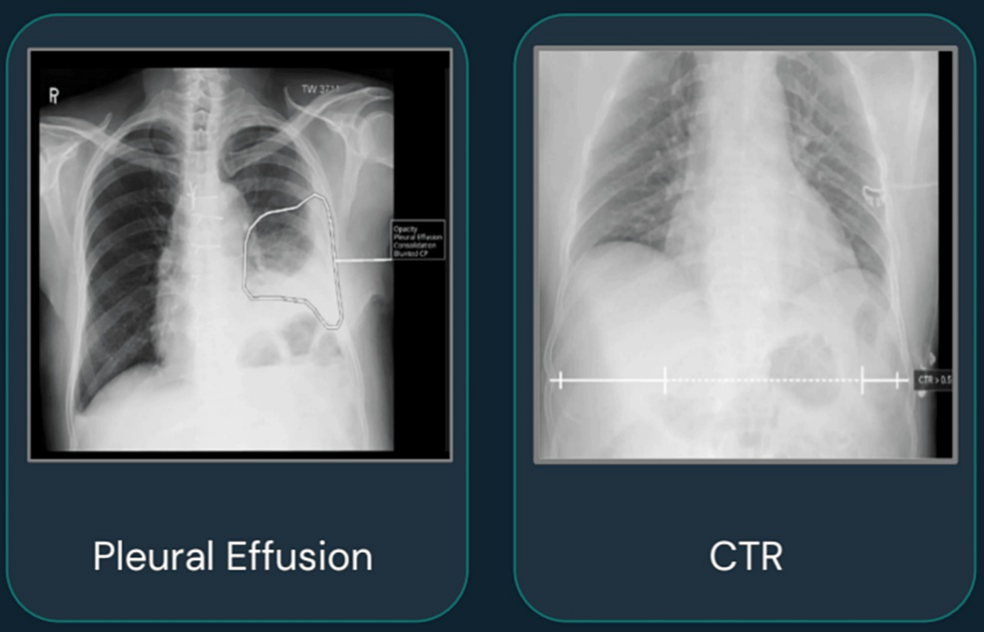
X-ray images with Qure.AI software in heart failure Image credits: Permission was obtained from the original publisher to adapt this image [16]. CTR: Cardiothoracic ratio.
